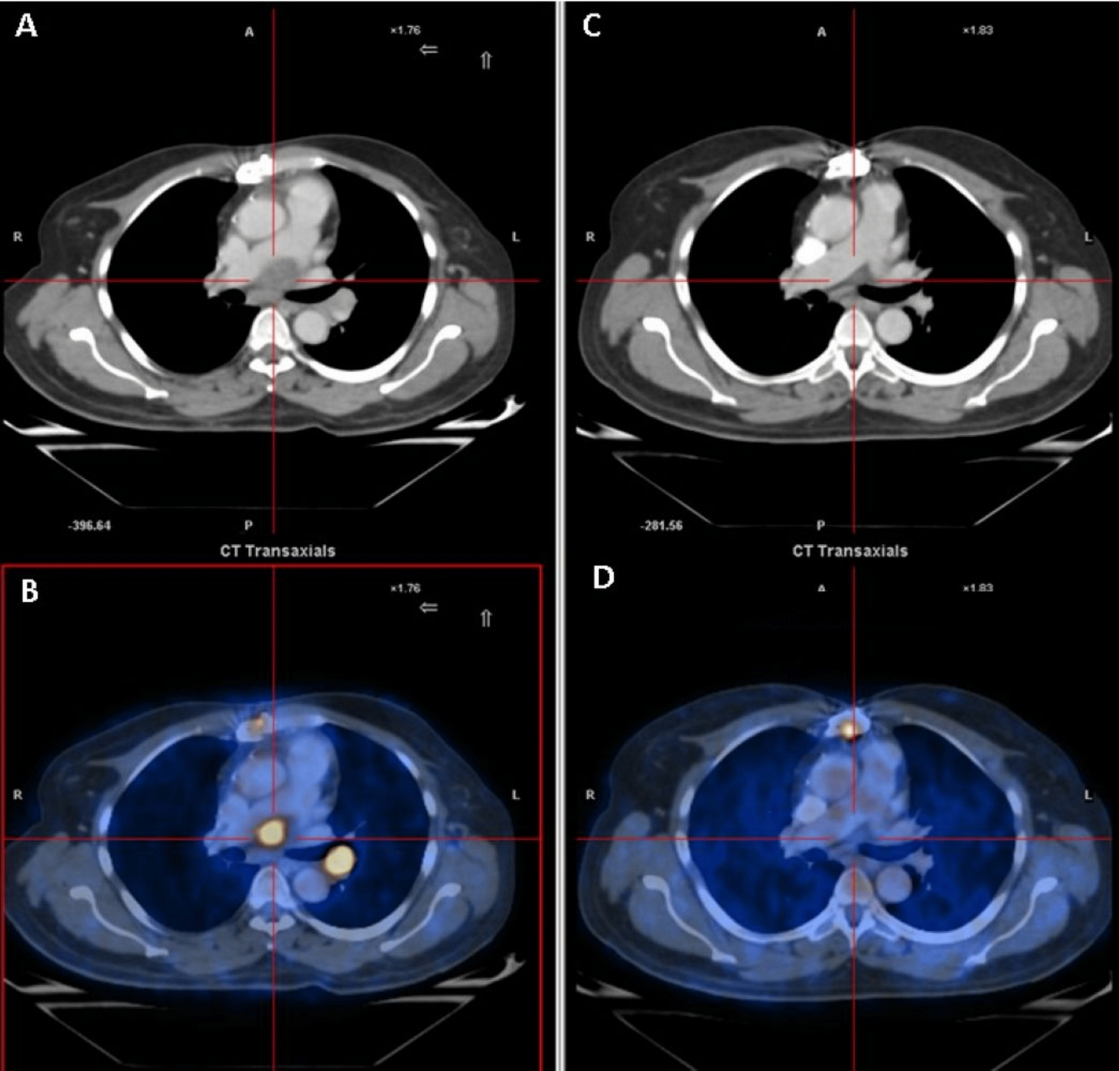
Comparison of PET-CT imaging before and after chemotherapy. Axial CT image from the postoperative PET-CT showing soft-tissue thickening along the interatrial septum (A). Corresponding fused PET-CT image demonstrating intense FDG uptake at the same site (B). Axial CT image after four cycles of R-CHOP chemotherapy showing marked reduction of the lesion (C). Corresponding fused PET-CT image demonstrating near-complete resolution of metabolic activity (D).
